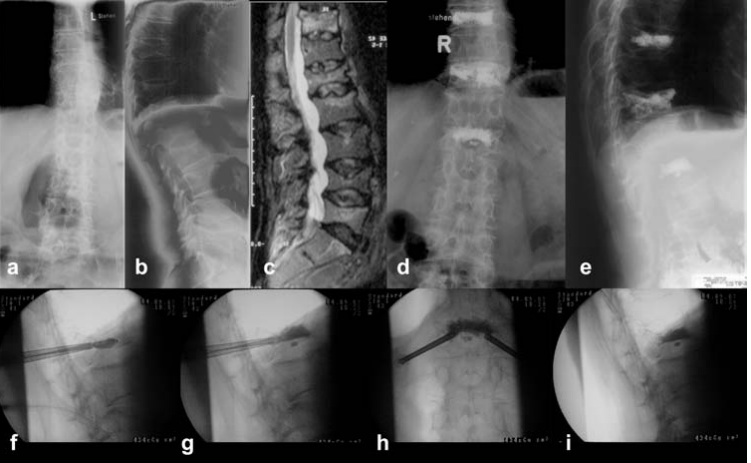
A 77-year old man complained about severe thoracolumbar back pain. Plain radiographs revealed multiple osteoporotic vertebral compression fractures (a, b), of which fractures at Th9, Th11, and L1 were relatively fresh in the stir-sequence of the MRI (c). Because of severe pain resistant to non-surgical therapy for 2 months we decided to perform kyphoplasty at Th9, Th11, and L1. During the procedure the flattened vertebra L1 was impossible to reduce (f), while filling the vertebra with PMMA cement a leakage occurred into the lower disc (g-i). Nevertheless the patient had dramatically reduced back pain, presented no sign of neurological damage and was released two days after the procedure (d, e).
